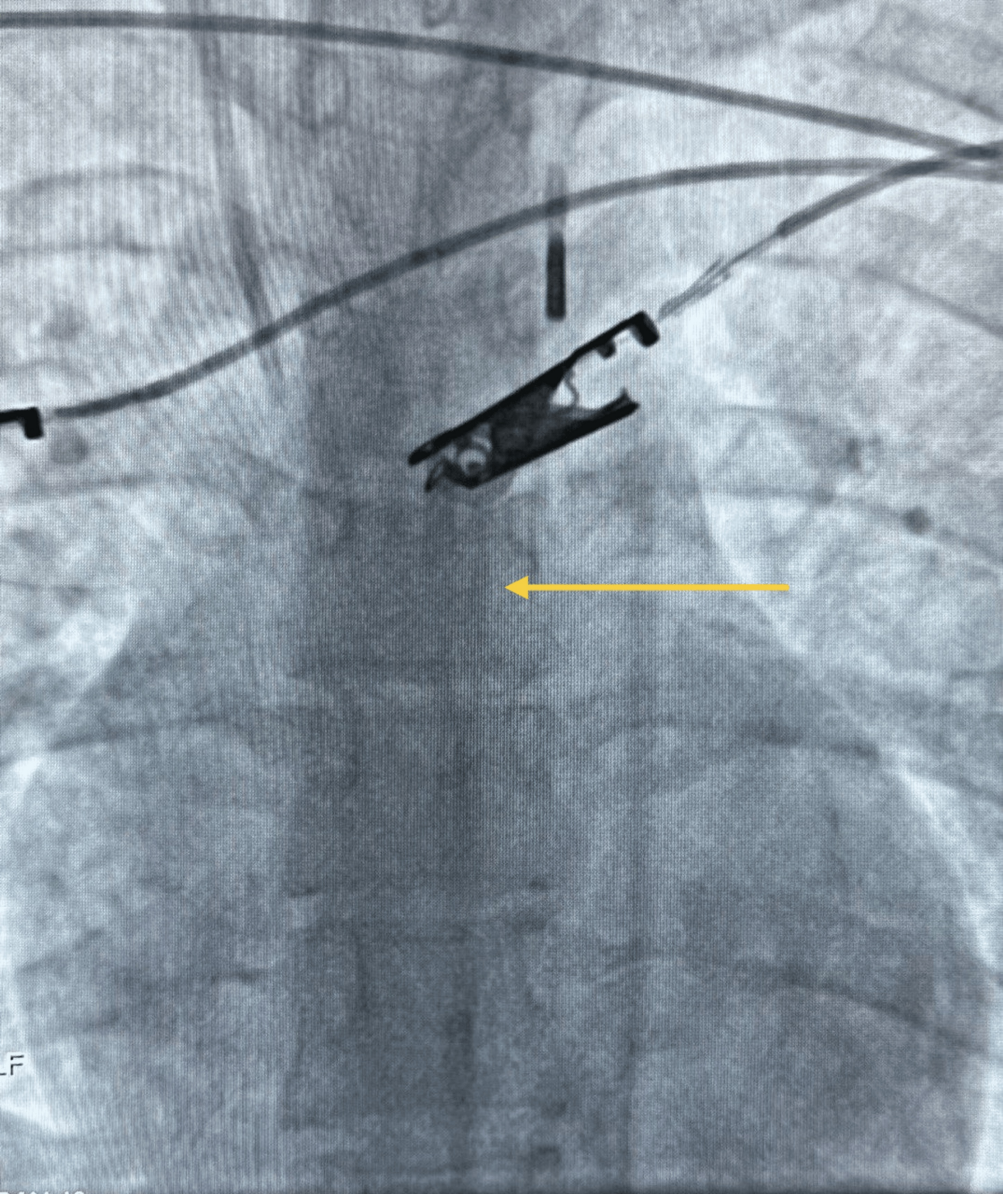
Chest X-ray showing IABP insertion in the patient’s aorta IABP, intra-aortic balloon pump
